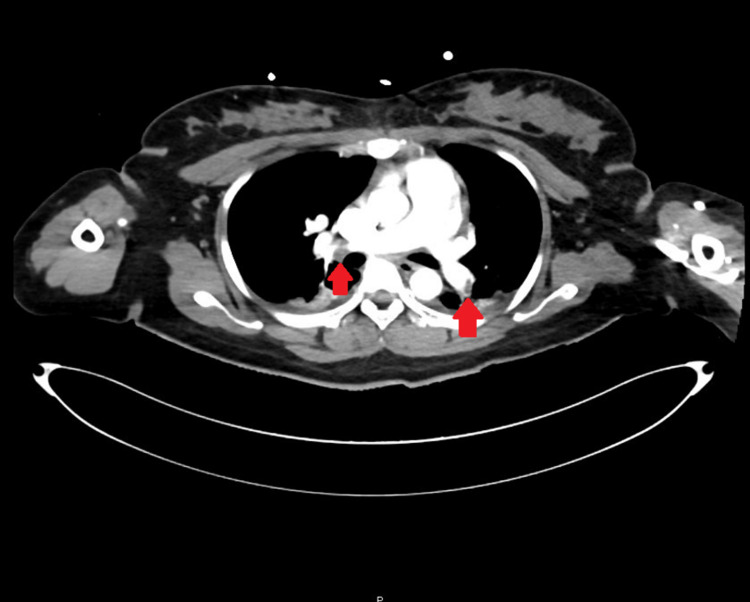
CT pulmonary angiography showing bilateral pulmonary embolism
